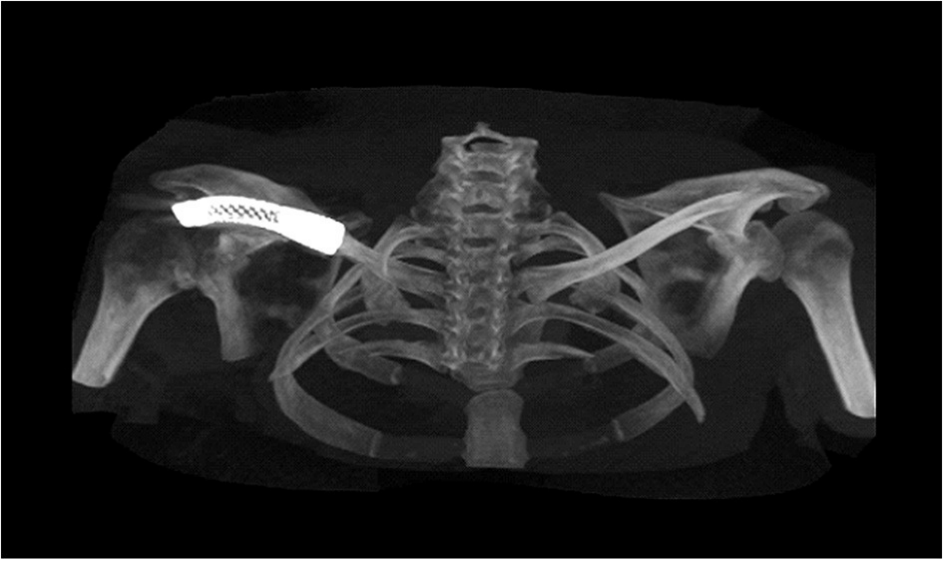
Postoperative computed tomography scan 6 months after the operation revealed a good position for the prosthesis and no evidence of a stress fracture.
